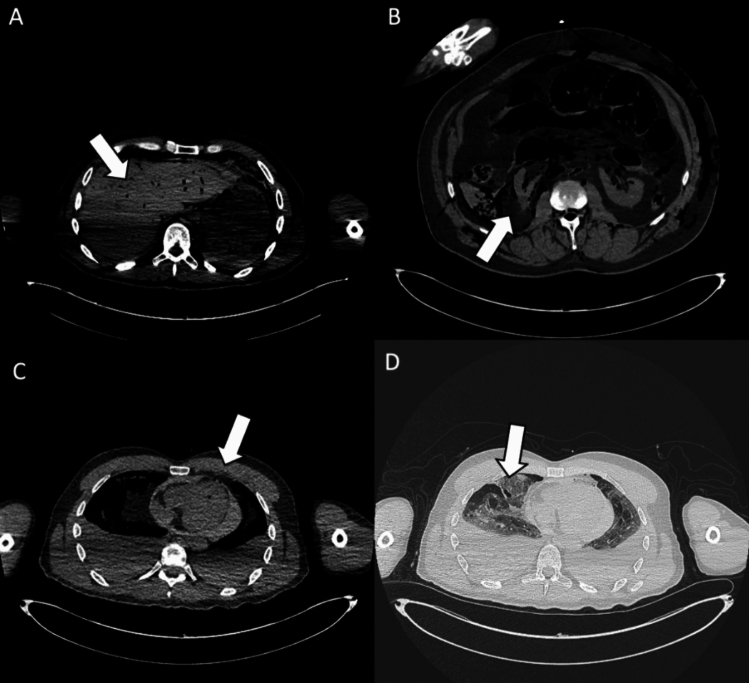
Organ injuries. A Axial CT scan showing traumatic injury to the bi-lobar liver parenchyma (white arrow). Moderate postmortem decomposition is also evident, with heterogeneous intraparenchymal gas distribution. B Cross-sectional CT image demonstrating a laceration of the right kidney (white arrow), consistent with penetrating trauma. C Axial CT slice showing a pericardial lesion (white arrow), with small gas bubbles in the anterior pericardial region, indicative of traumatic pericardial injury. D CT image highlighting a laceration of the right middle lobe (white arrow), associated with pulmonary contusion, omolateral pneumothorax, and bilateral pleural effusion. In the assessment of organ injuries, inter-reader agreement was only moderate (Fleiss’ *k* = 0.52). Sensitivity was again limited among less forensic-experienced readers (31.0% and 51.7%), reflecting the interpretative complexity and reduced diagnostic accuracy of PMCT in this setting
